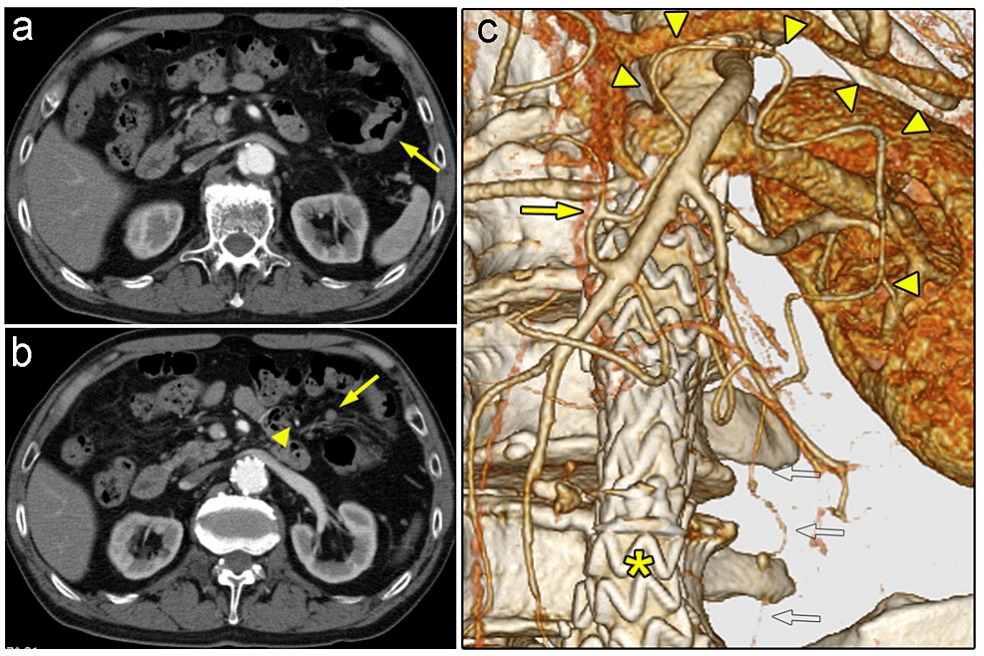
Preoperative images for case one a) A CT scan showing thickening of the wall at the splenic flexure (arrow). b) An enlarged regional lymph node (arrow) was detected between the tumor and the arterial arcade (arrowhead). c) CT angiography demonstrated that the left branch of the MCA (arrow) was anastomosed to the LCA via the arterial arcade (arrowheads). There was no sign of blood flow at the root of the IMA, and the SRA (open arrows) was barely patent because of aortic stenting (asterisk). CT: computed tomography; IMA: inferior mesenteric artery; LCA: left colic artery; MCA: middle colic artery; SRA: superior rectal artery
